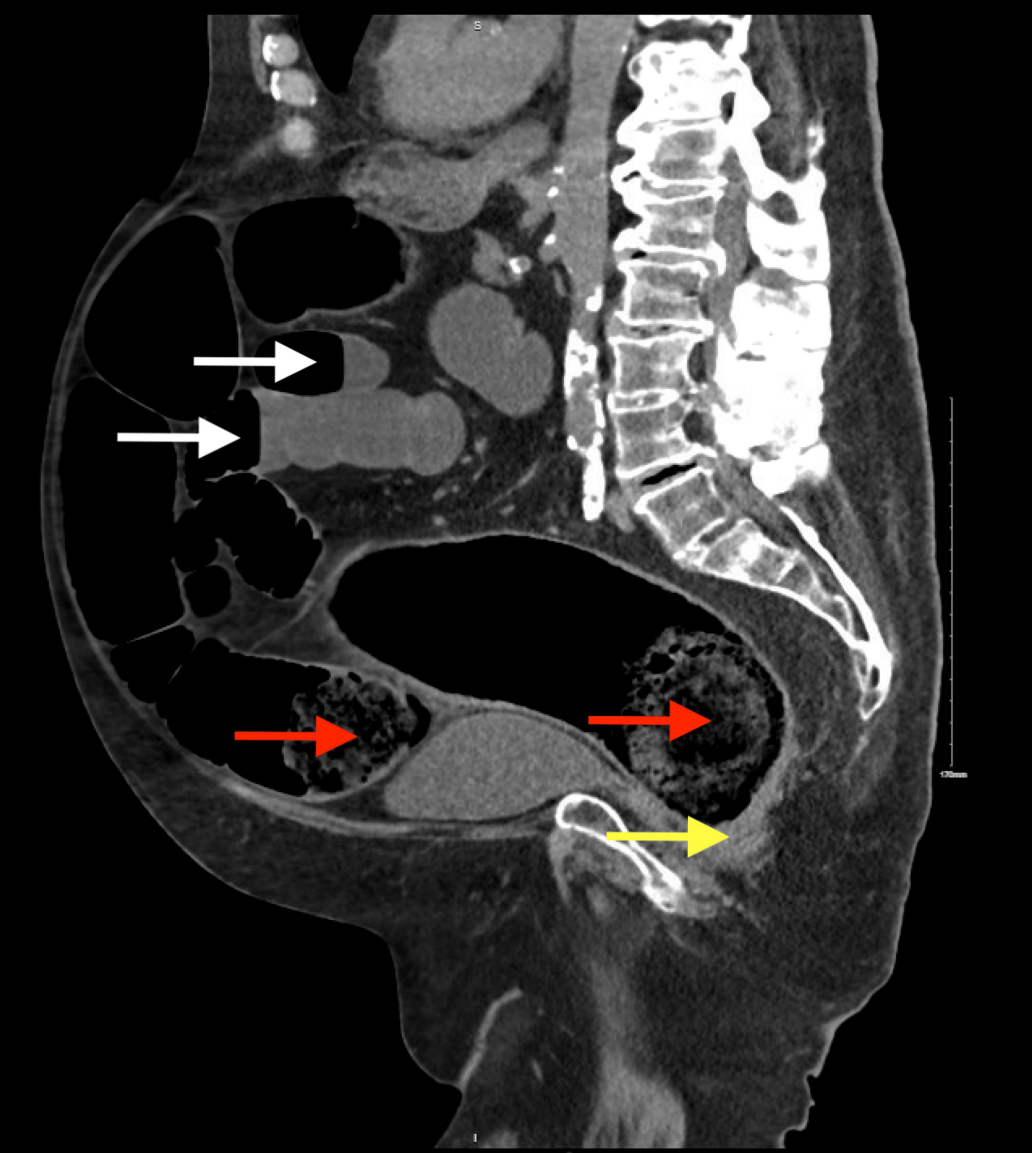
Computed tomography scan of the abdomen Computed tomography scan of the abdomen on hospital day 1 demonstrating severe fecal impaction (red arrows), stercoral colitis (yellow arrow), and multiple dilated loops of small bowel with air-fluid levels (white arrows).
